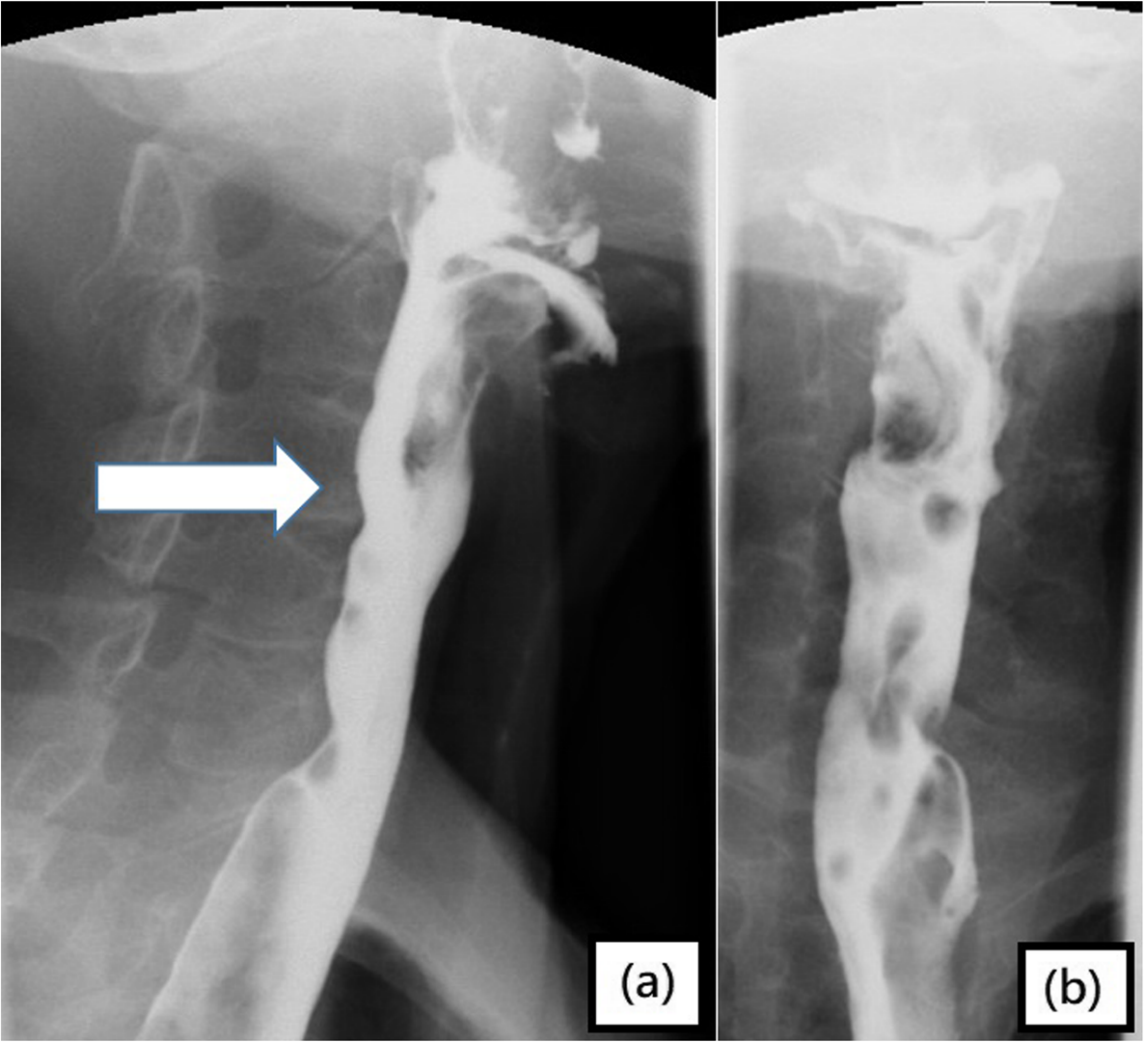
Dilatation of proximal cervical segment was noted on: a lateral and b anteroposterior radiographs of barium radiographic test* after cricopharyngeal myotomy
